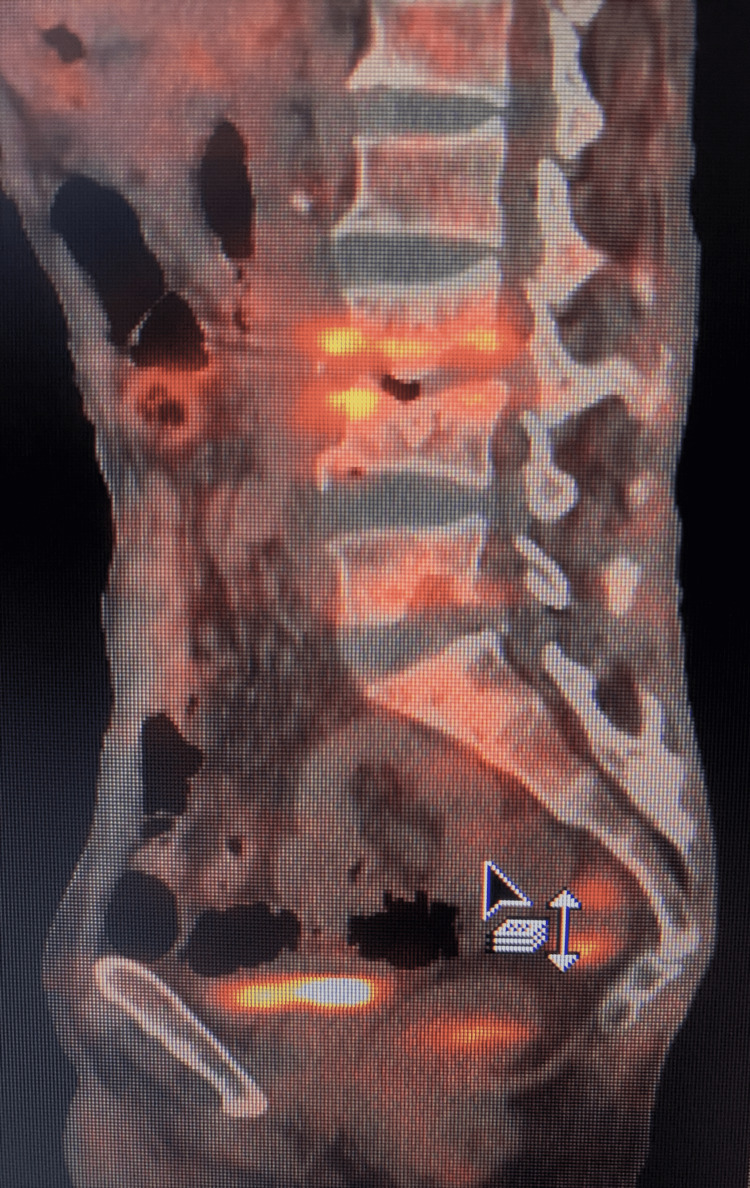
FDG PET/CT of the spine FDG PET/CT showing degenerative changes of the spine along with the collapse of L3 vertebra, end plate changes with the erosion of superior end plate of L4 and inferior end plate of L3 vertebra Increased FDG uptake was seen in L3 - L4 intervertebral disc (SUVmax = 6.3) suggestive of residual disease. FDG - fluorodeoxyglucose, PET - positron emission tomography, SUVmax - maximum standardized uptake value
